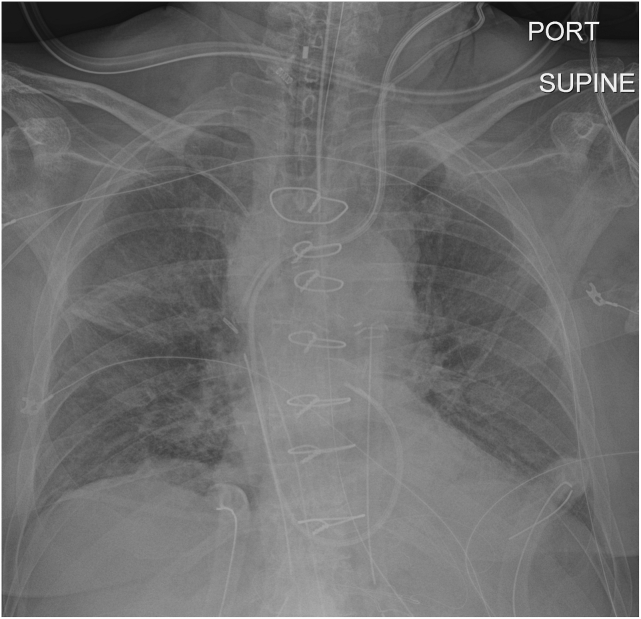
Radiograph of the patient’s chest on postoperative day 0 prior to extubation on postoperative day 1.
